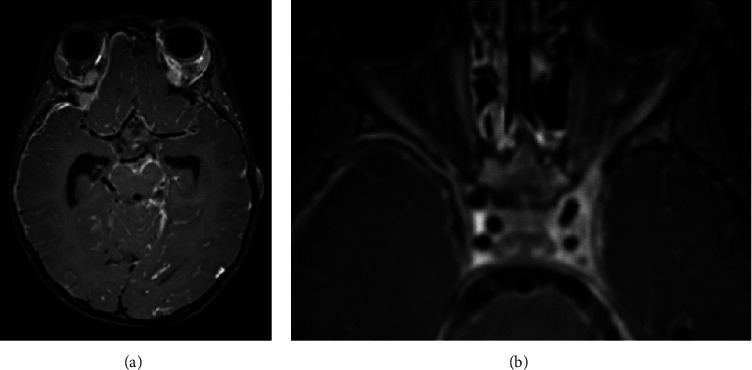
MRI day 1. Vessel wall imaging (axial 3D T1 TSE SPACE Dante) after IV gadolinium administration. (a) There is diffuse supratentorial and infratentorial pathological leptomeningeal contrast enhancement compatible with meningitis. Also note the pathological contrast enhancement of the walls of the cerebral arteries, indicative of associated vasculitis. (b) The cavernous sinus appears thickened on the left side and enhances more heterogeneously, suggestive of inflammation. There is secondary narrowing of the left cavernous carotid artery.
